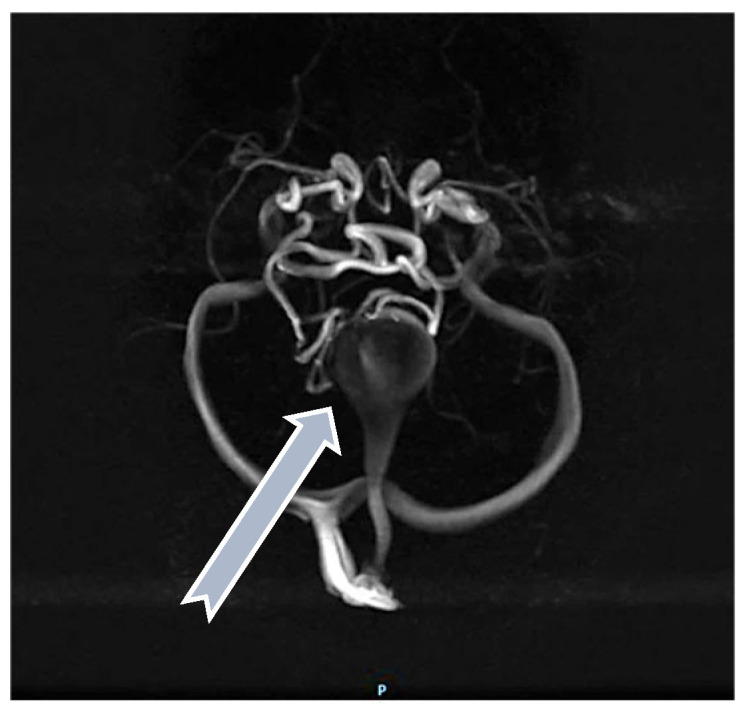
Angio-MRI sequence: Vascular aneurysmal dilation located at the level of the quadrigeminal tank (blue arrow), communicating with the large cerebral veins and the left middle cerebral artery, with collaterals emerging from the choroidal and posterior cerebral arteries—highly suggestive aspect of Galen vein aneurysm.
